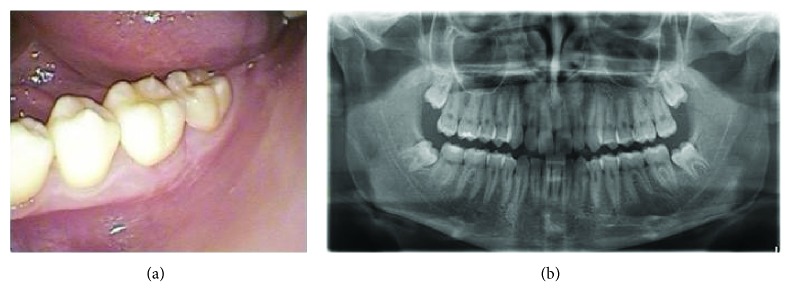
(a) Clinical image: excellent scarring of the area where the drainage catheter was placed. (b) Control orthopantomography 9 months after surgery. Significant reductions are seen in the size of the lesion and in the presence of cancellous bone.
